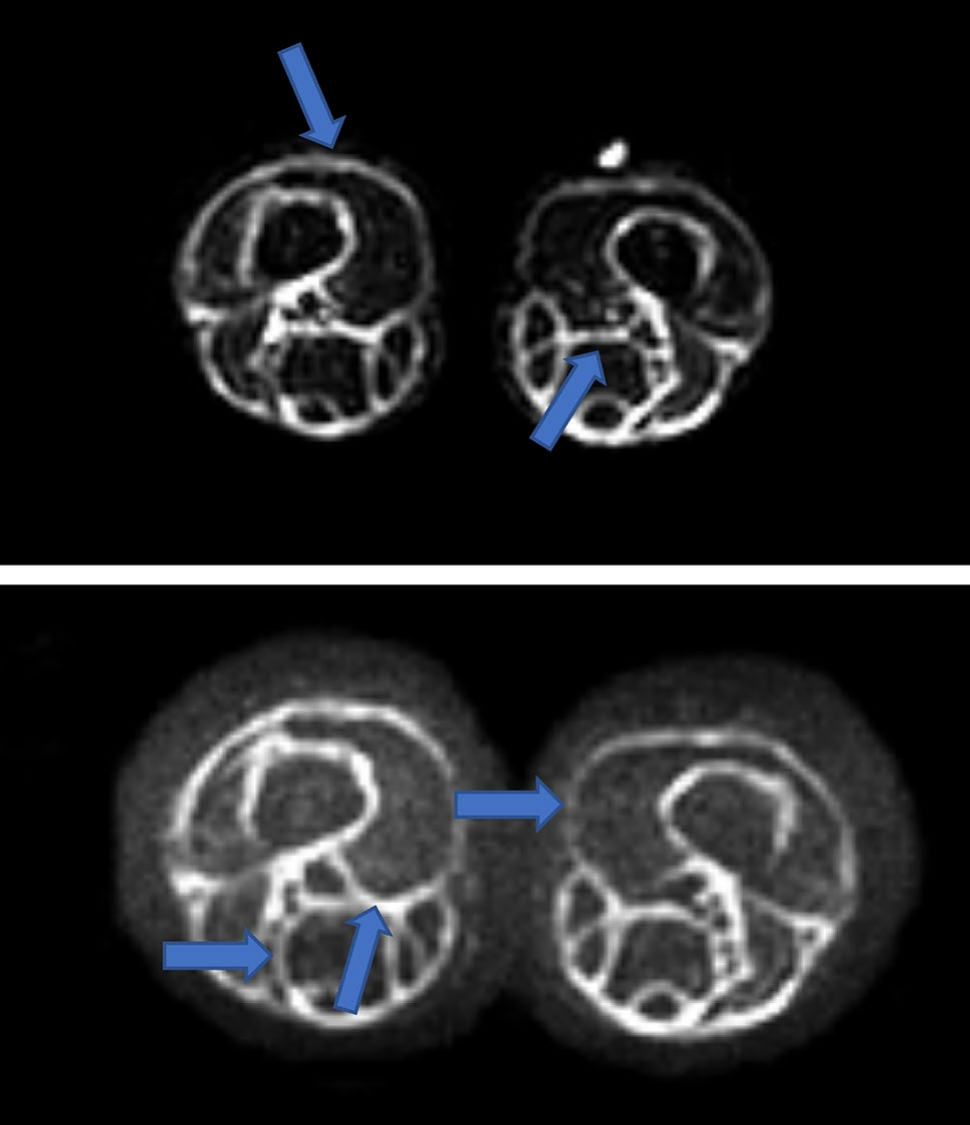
Hyperintense signal of fascia of lower limbs showed in MRI (highlighted by arrows)
